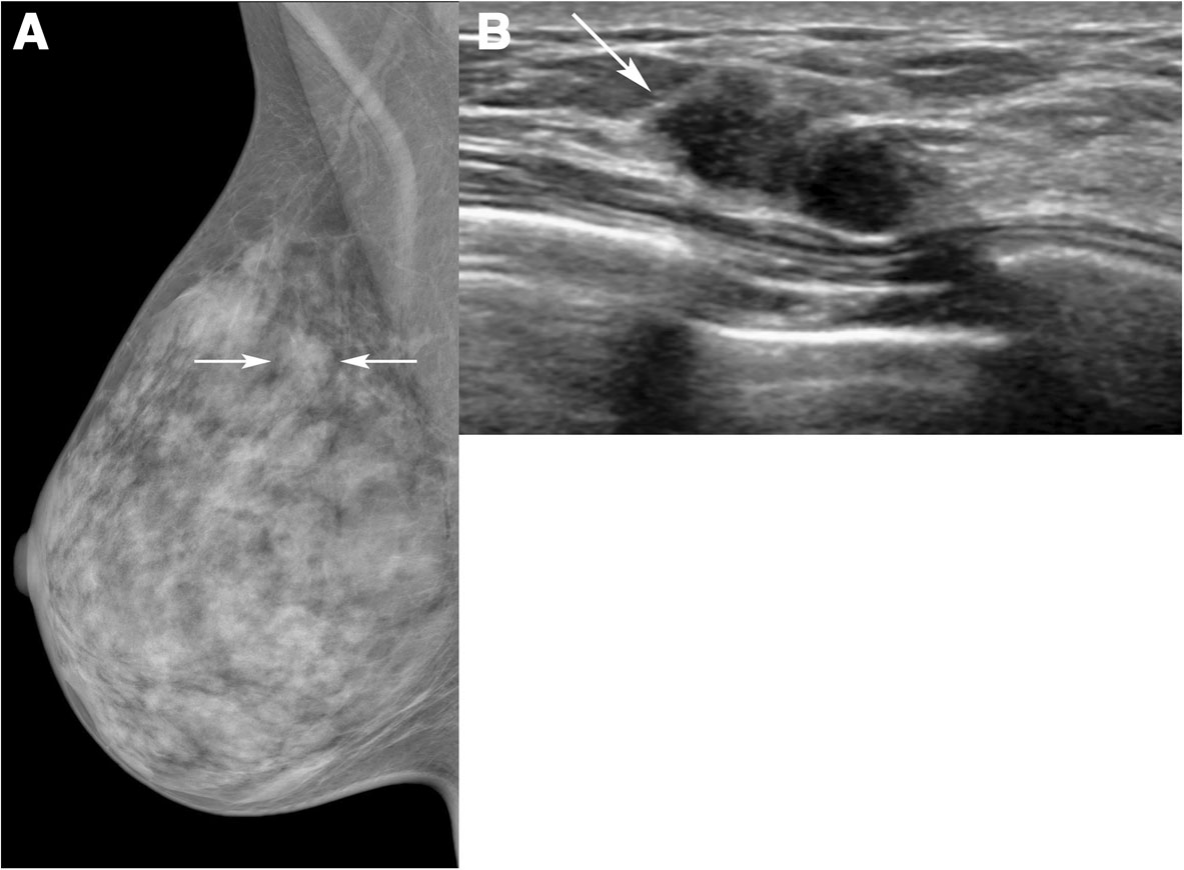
A 39-year-old woman with a PHBC in the left breast, classified as interpretation error, ‘subtle finding’. a Mediolateral oblique mammogram shows an asymmetry (arrows) on the right upper breast. b Ultrasound image shows a 15-mm irregular hypoechoic mass (arrow) in the right breast, which was pathologically proven to be an invasive ductal carcinoma
